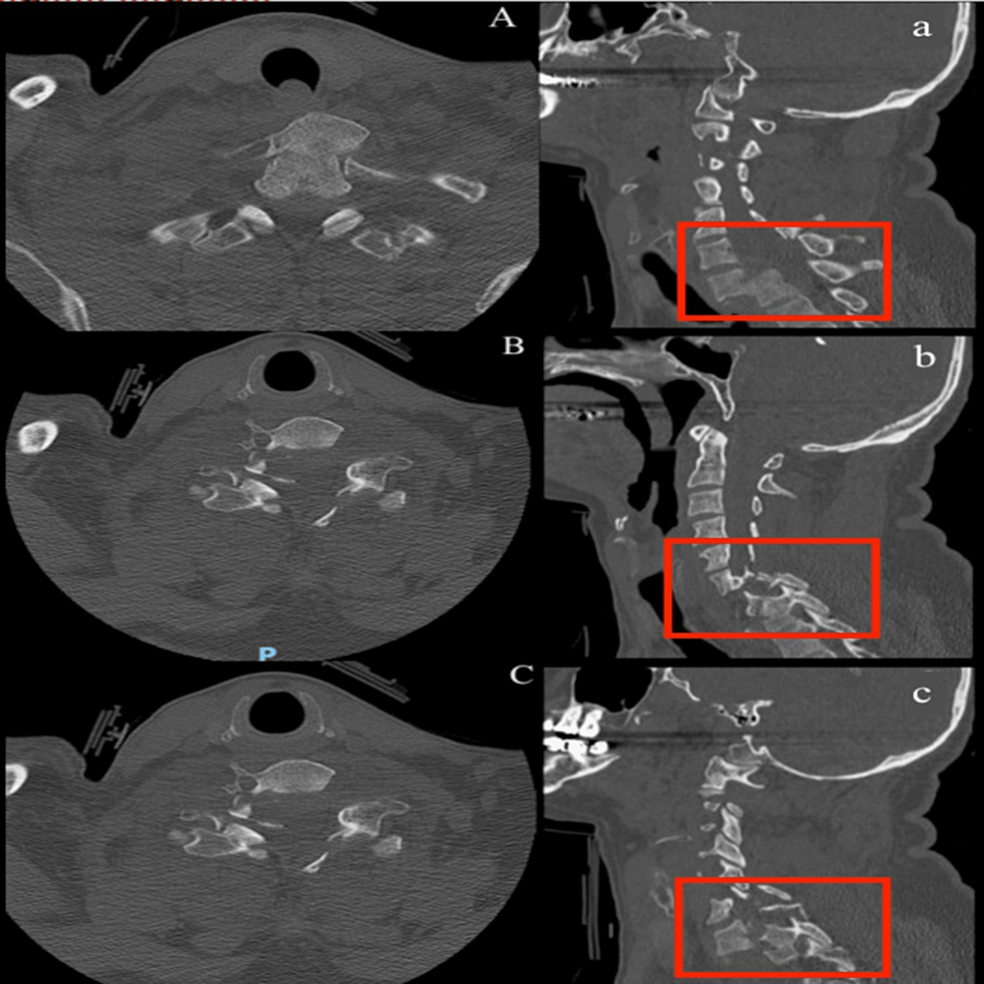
Preoperative computed tomography scan. Sagittal (a,b,c) and axial (A, B, C) views of computed tomography scan showing midcut (A,a), right (B,b), and left (C,c) facet fracture dislocation.
